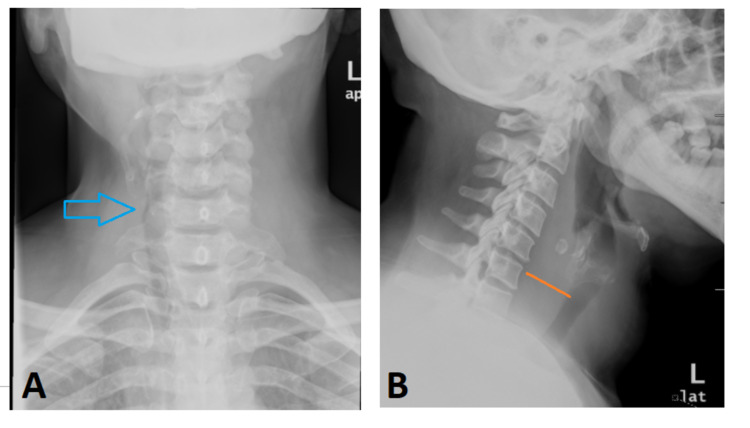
X-ray neck. (A) Anteroposterior view showed soft tissue mass seen mainly on the left side of the neck causing significant upper trachea deviation to the right side (arrow head). (B) Lateral view showed mass was extending from the anterior compartment to posterior compartment of the neck with abnormal widening of anterior paravertebral space (Orange line).
